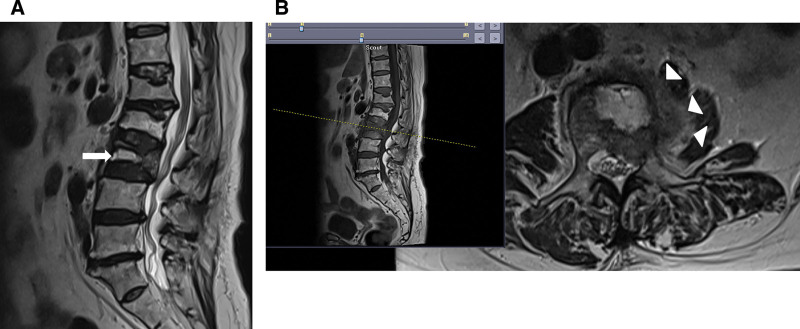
Non-contrast magnetic resonance imaging of the lumbar spine. (A) Sagittal T2-weighted image showing the collapse of the L3 vertebral body with an intravertebral fluid cleft (arrow). (B) Sagittal T1-weighted and axial T2-weighted images demonstrating paravertebral soft tissue edema accompanied by fluid collection (arrowheads).
